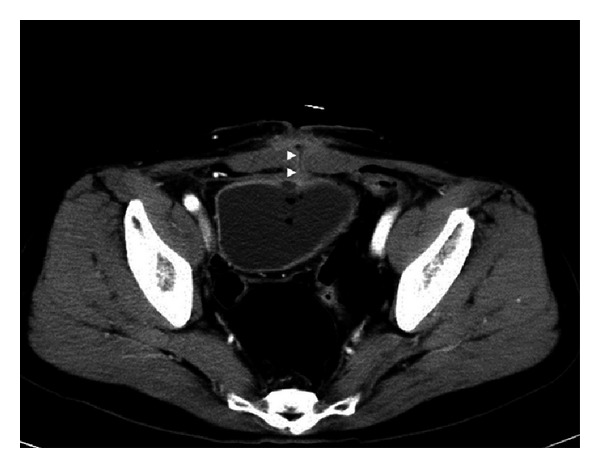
Axial contrast-enhanced computed tomography images of the abdomen showing a fistula (arrowheads) between the neobladder and skin, without apparent ischemia of the neobladder ileum.
